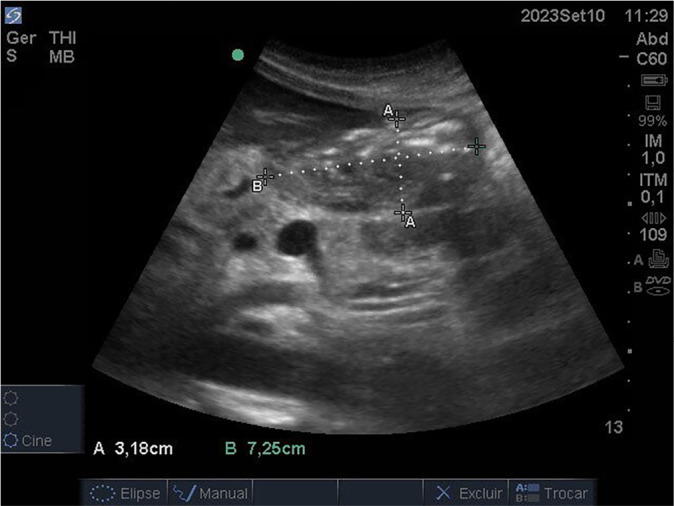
Gastric antrum ultrasound image on intensive care unit day 27
